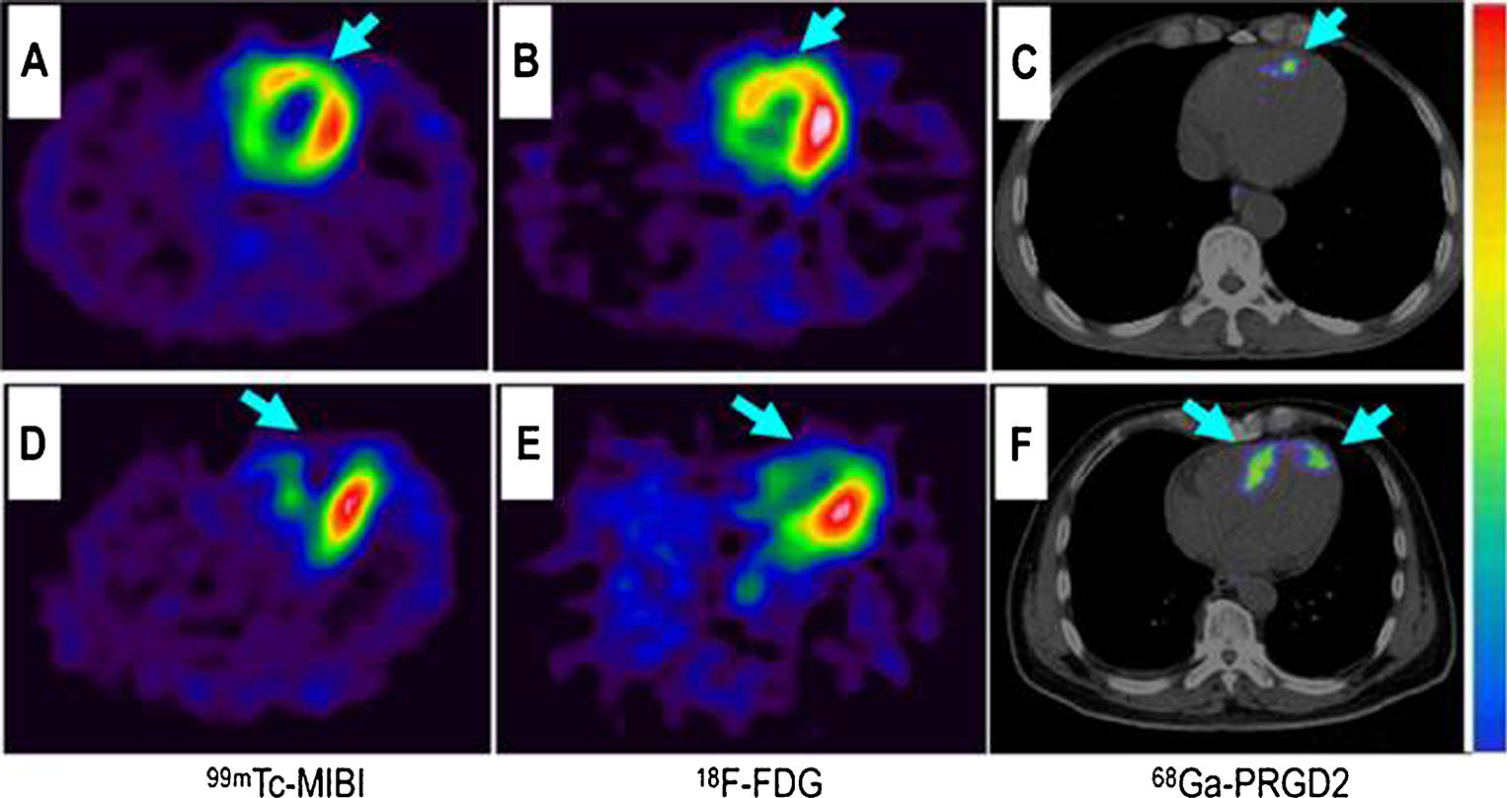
Comparison of a patient with slight myocardial infarction (MI) and a patient with severe MI. *Upper row*: In a 58-year-old man at the fifth day after the event, a small apical region with decreased 99mTc-MIBI perfusion (a, *arrow*) and 18F-FDG metabolism (b, *arrow*) showed mild 68Ga-PRGD2 accumulation (c, *arrow*), with a pSUV of 0.62. *Lower row*: In a 45-year-old woman on the seventh day after the event, an apical defect on 99mTc-MIBI perfusion images (d, *arrow*) and 18F-FDG metabolism images (e, *arrow*) corresponded with moderate 68Ga-PRGD2 uptake (F, *arrows*), with a pSUV of 2.02 (reprinted from [55••] with permission from Theranostics)
